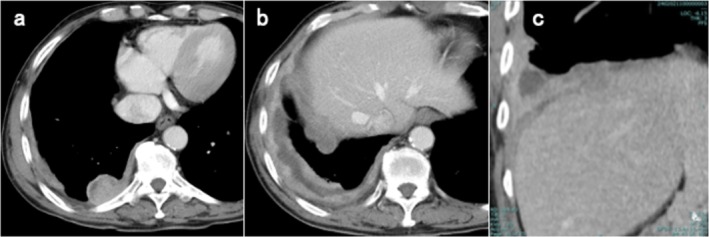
Contrast‐enhanced computed tomography images obtained 30 days before nivolumab plus ipilimumab administration. (a) Shows a pleural‐based mass in the dorsal segment of the right lower lobe; (b) shows pleural thickening, effusion, and multiple masses at the right lung base. (c) A coronal reconstruction of (b), showing blurring of the medial right hemidiaphragm, suggestive of liver invasion.
